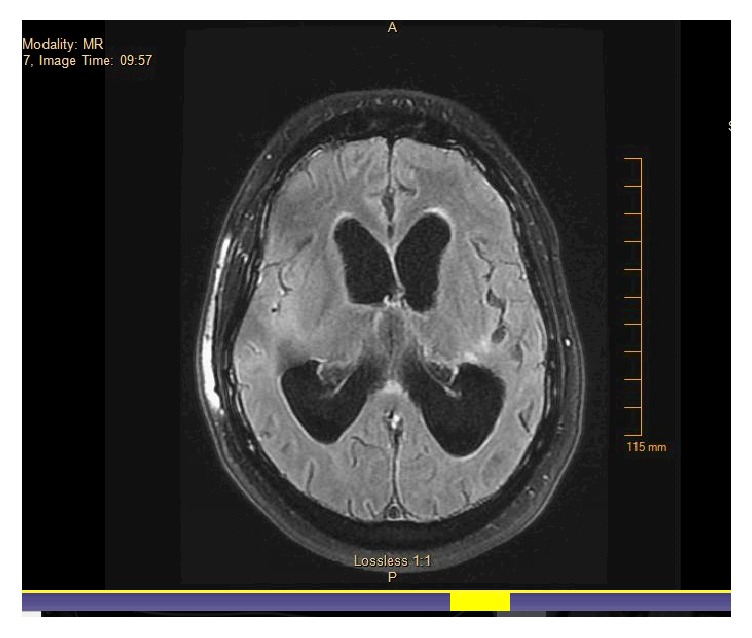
Post-rituximab MRI did not show any significant interval change in findings suggesting leptomeningitis/pachymeningitis, and foci of prolonged T2 values within the white matter.
